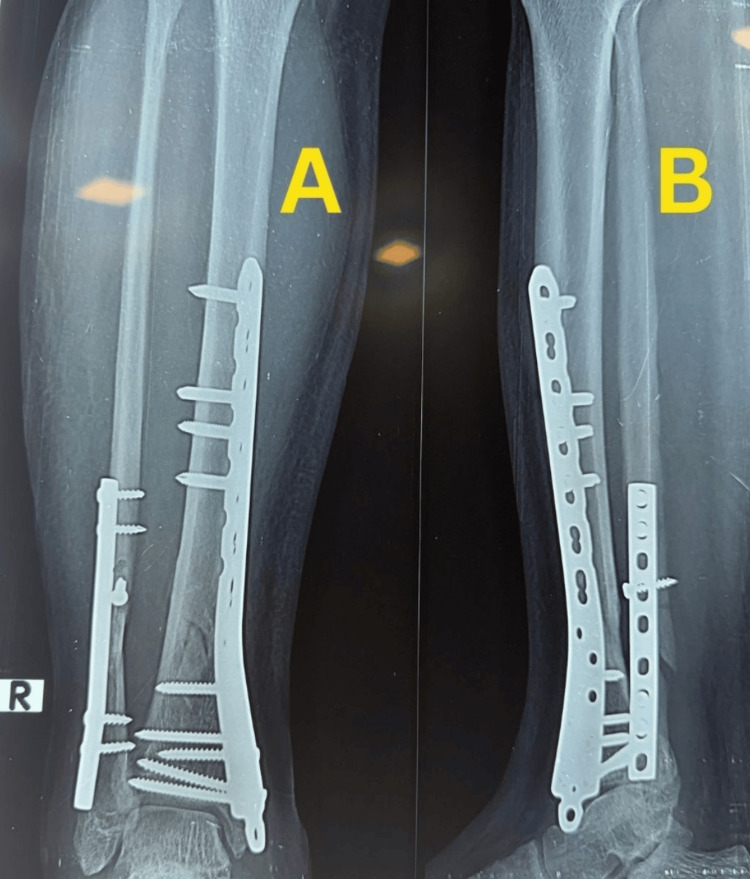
Final follow-up radiographs of Case 1. (A) Anteroposterior view showing complete fracture union with good alignment and remodeling, with the implants in situ. (B) Lateral view confirming solid union of the distal tibia.
